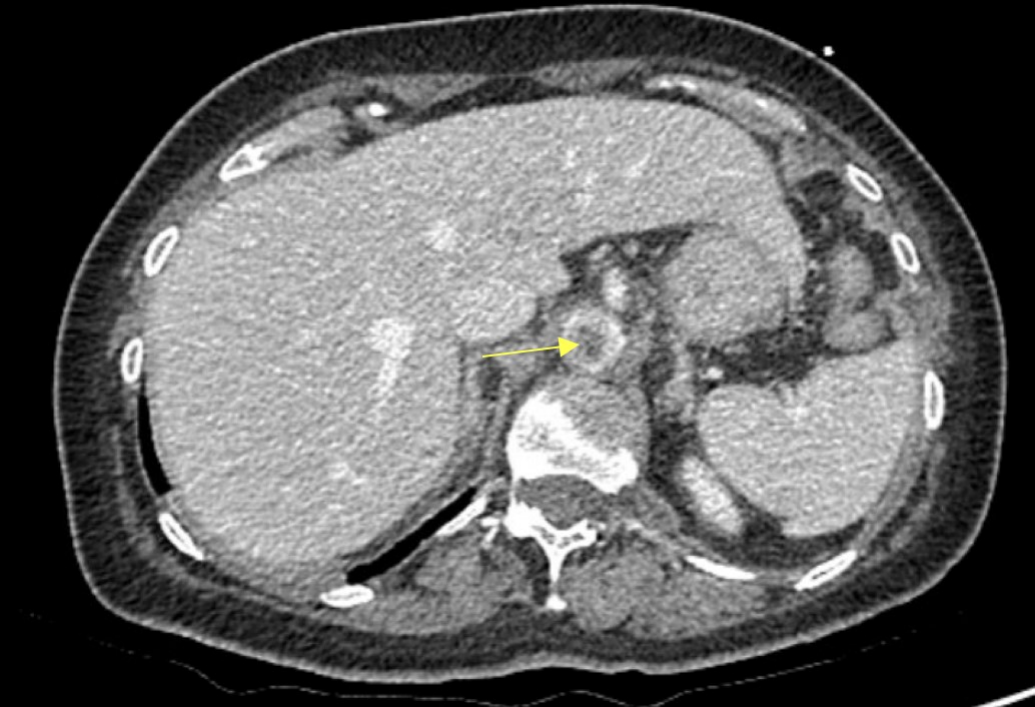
Computed tomography image showing an abdominal aortic thrombus (yellow arrow).
